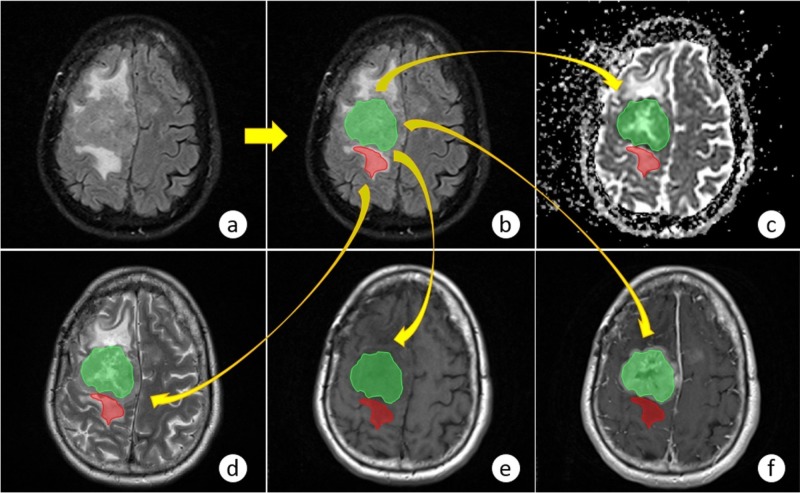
Segmentation process applied to the five different MRI sequences used in the study: (a) original image; (b) FLAIR; (c) ADC; (d) T2-W and DCE; (e) precontrast and (f) postcontrast ADC: apparent diffusion coefficient; DCE: dynamic contrast-enhanced; FLAIR: fluid-attenuated inversion recovery; MRI: magnetic resonance imaging; T2-W: T2-weighted
